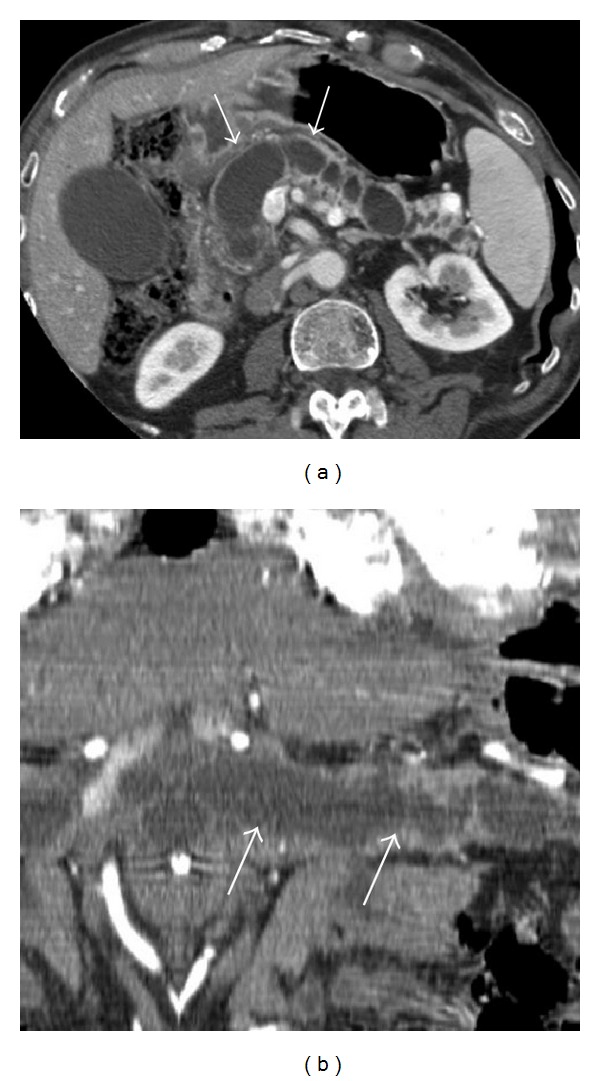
CT postcontrast examination in a patient who was suffering from jaundice and abdominal pain: axial images after contrast administration (a) and curved-MPR images (b). White arrows in (a) and (b) show a marked dilatation of the entire main pancreatic duct, from the head to the tail of the gland, associated with subtotal parenchymal atrophy. No dilatation of secondary branches was observed, and radiological diagnosis of MD-IPMN was formulated. The high degree of main pancreatic duct dilatation (>1 cm) was considered as high-risk stigmata and required surgical treatment. In addition, white arrows show mild wall enhancement. Final diagnosis of invasive cancer (adenocarcinoma) in IPMN was reported.
